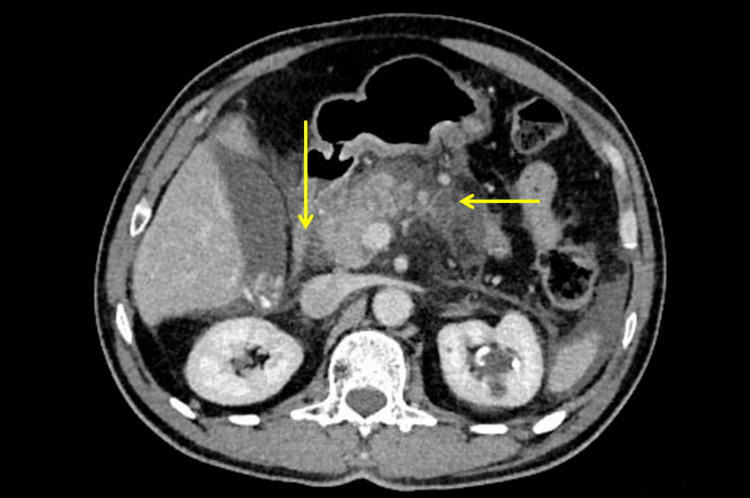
Abdominal contrast-enhanced computed tomography showing pancreatic necrosis and peripancreatic fat stranding (yellow arrows).
